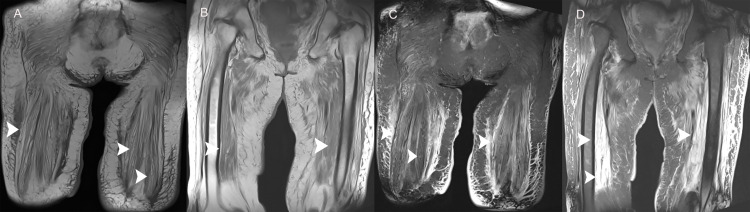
Coronal T1-weighted and coronal fat suppressed T2-weighted images of the pelvis and the thighs. Coronal T1-weighted images of the pelvis (a) and the thigh (b) show diffuse muscle atrophy. Coronal fat suppressed T2-weighted images of the pelvis (c) and the thighs (d) show diffuse muscle atrophy and hyperintense signal changes indicating muscle edema and inflammation (arrowheads).
